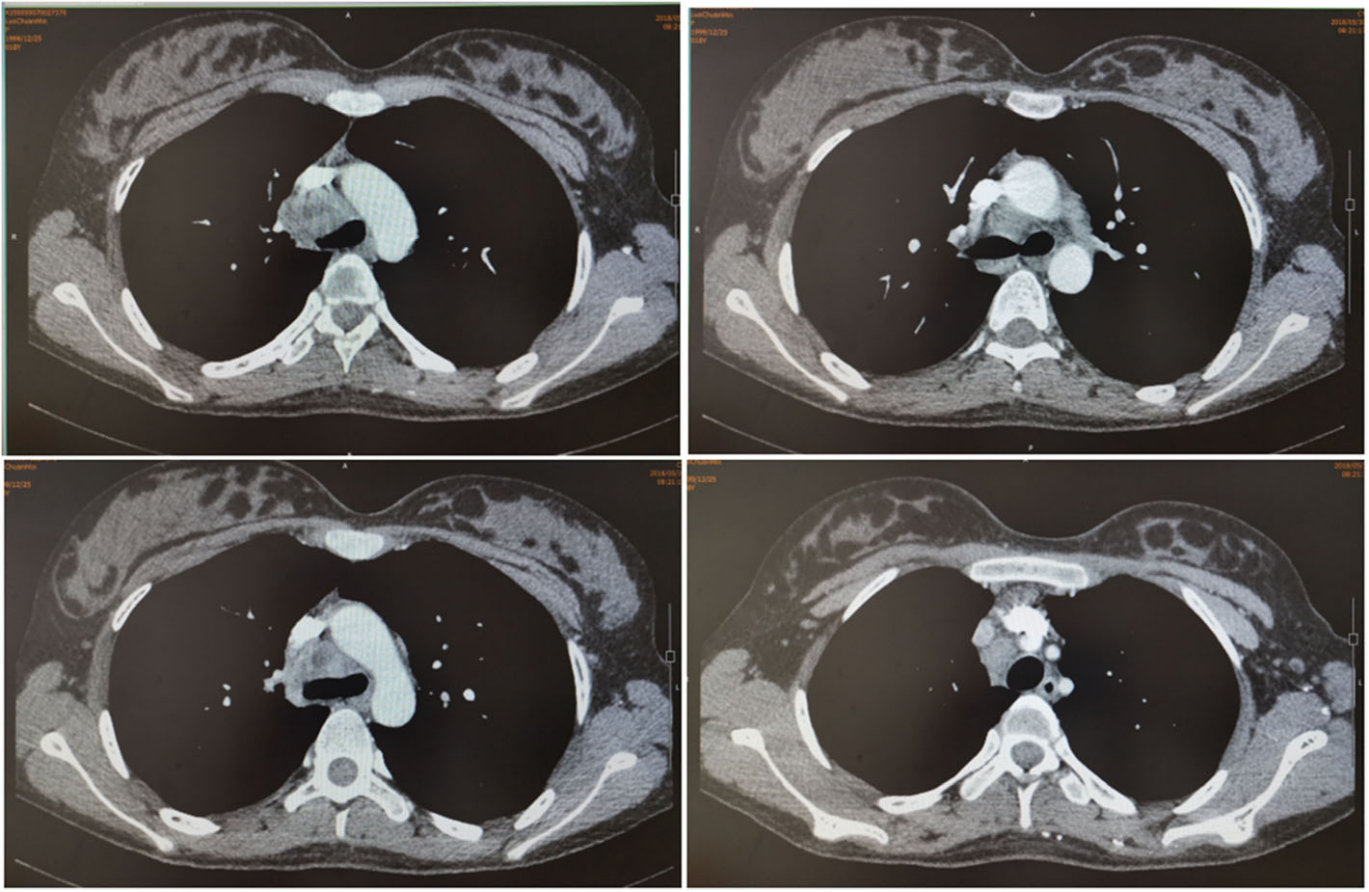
Chest CT reexamination on May 30, 2018
